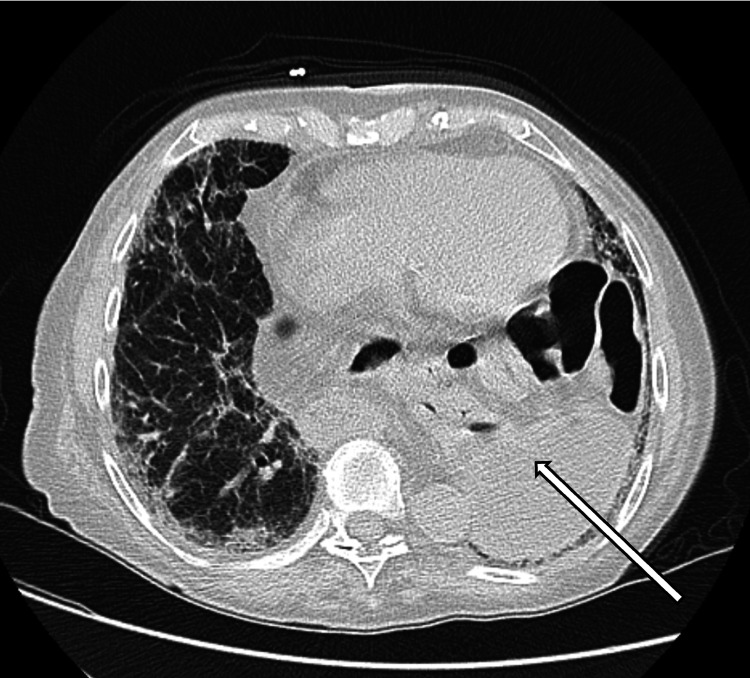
CT Chest Coronal view in the lung window showcasing the herniation of the stomach and bowel into the left hemithorax.
